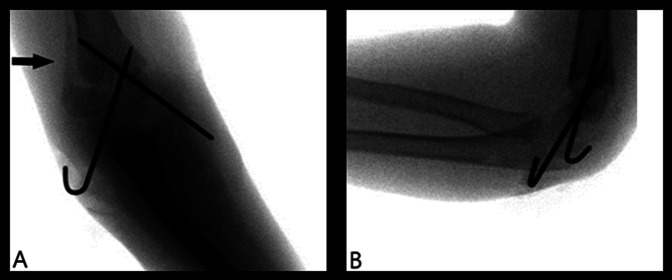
Postoperative radiographs showing cross-pin fixation of fracture at 4 weeks. A, Anteroposterior view shows exposed normal posterior lateral periosteal bone formation (arrow). B. Lateral view.
